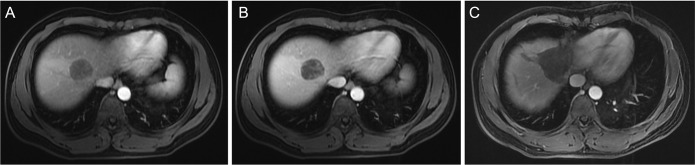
(A, B) Enhanced MR at 17 weeks post-bHAIC shows significant regression of the liver lesion following a single session of bHAIC treatment, with complete disappearance of lesion enhancement. (C) The patient underwent radical ablation for the liver lesion, and the enhanced MR image at 8 weeks post-ablation revealed that the necrotic area was slightly larger than the original lesion.
